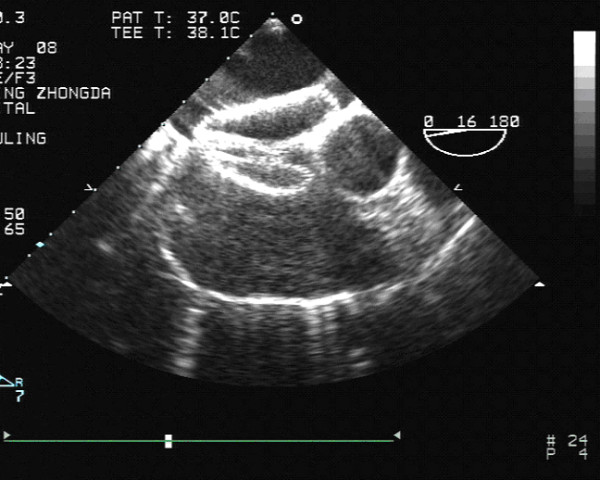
Transesophageal echocardiographic image of transcatheter ASD closure.
